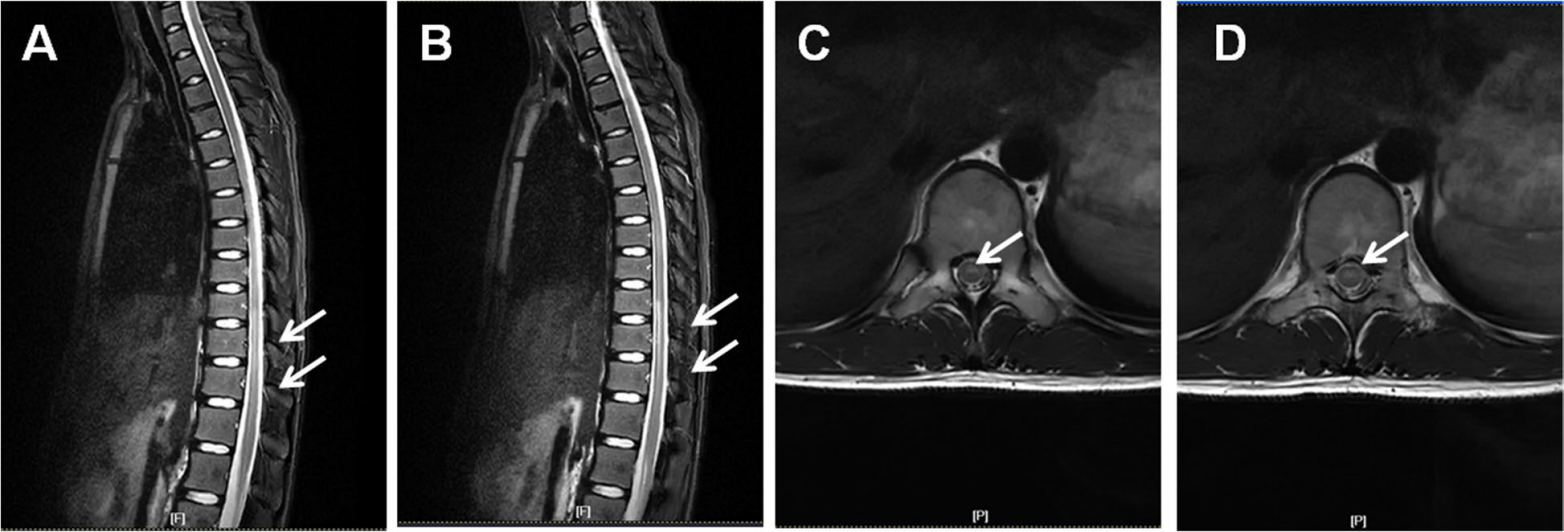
A, B The sagittal magnetic resonance imaging of the spinal cord in a 9-year-old patient with spinal cord injury (arrow). C, D Transverse section of the magnetic resonance image of the spinal cord injury (arrow)
